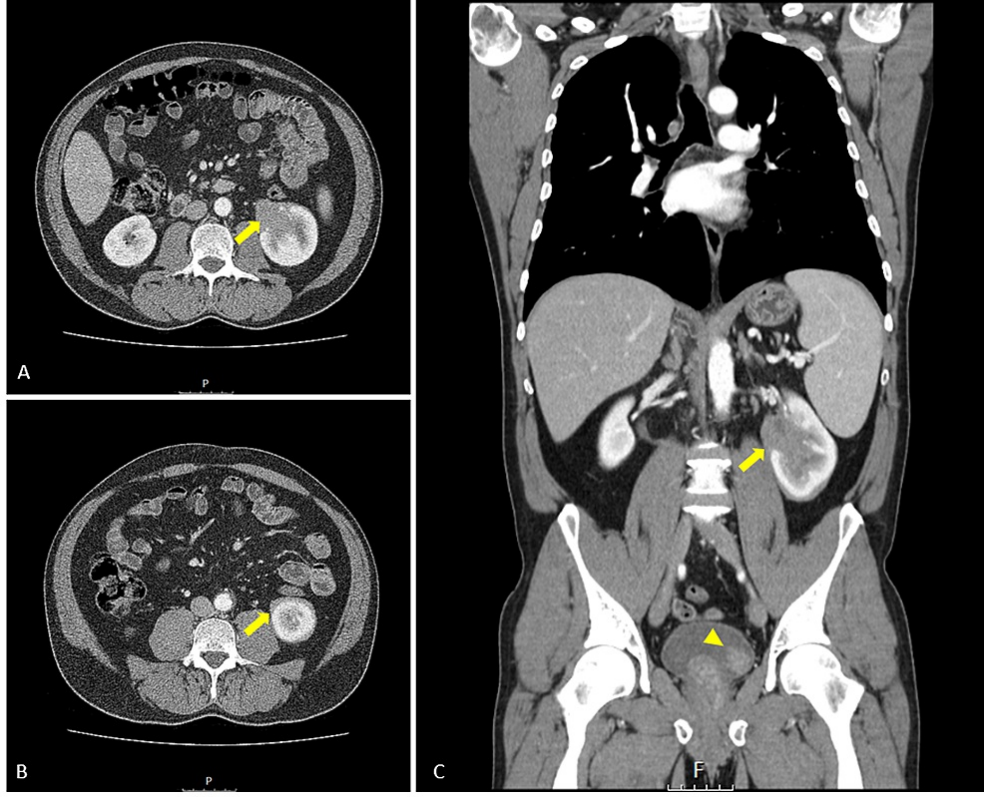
Axial (A and B) and coronal (C) CT scan Marked tissue densification in the renal sinus, suggesting marked thickening of the urothelium, conditioning deformity of the renal pelvis and calyces, particularly the middle and lower, with some heterogeneity of the renal parenchyma, highly suggestive of renal tumour (arrow); polypoid mass with 21 mm on the left lateral wall (arrowhead).
